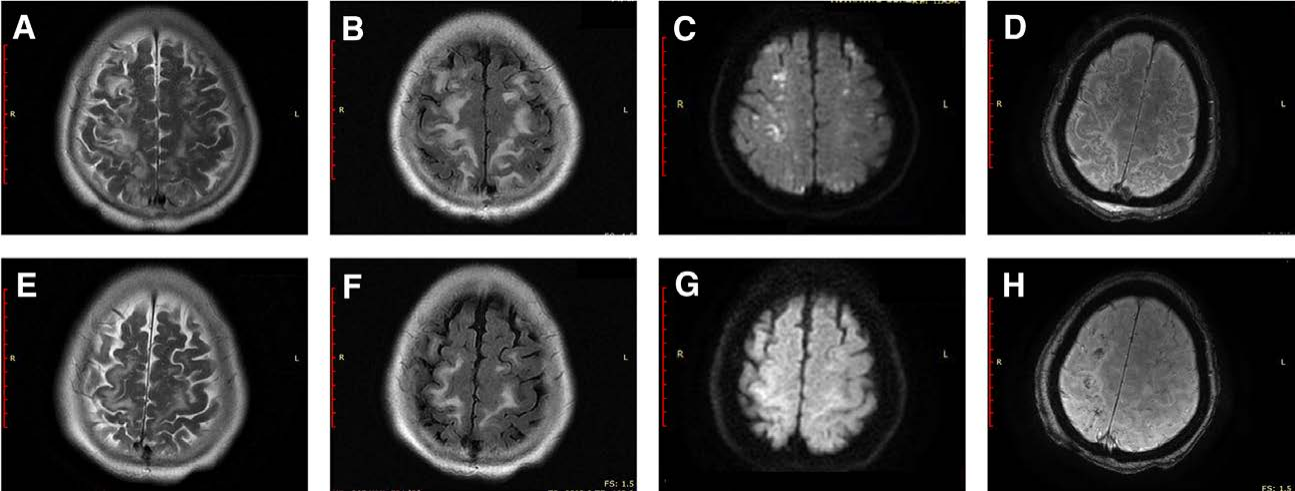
MR scans of the patient’s brain. T2W-MRI, FLAIR, DWI, and SWI sequences are shown from left to right: (A–D) are the images on the third day after onset, which showed extensive embolism lesions that spread along the bilateral cortices to the frontal, parietal cortex. Multiple pinpoint-like microhemorrhagic foci were seen in the lesion area, called “starry sky sign” on SWI. Images (E–H) were the reexamination images taken after 10 d. The scope of the lesions was smaller in (E–H) than 10 d before; microhemorrhagic lesions had a trend of fusion, and their overall number was reduced. DWI = diffusion-weighted imaging, FLAIR = fluid-attenuated inversion recovery, SWI = susceptibility-weighted imaging, T2 W MRI = T2-weighted magnetic resonance imaging.
